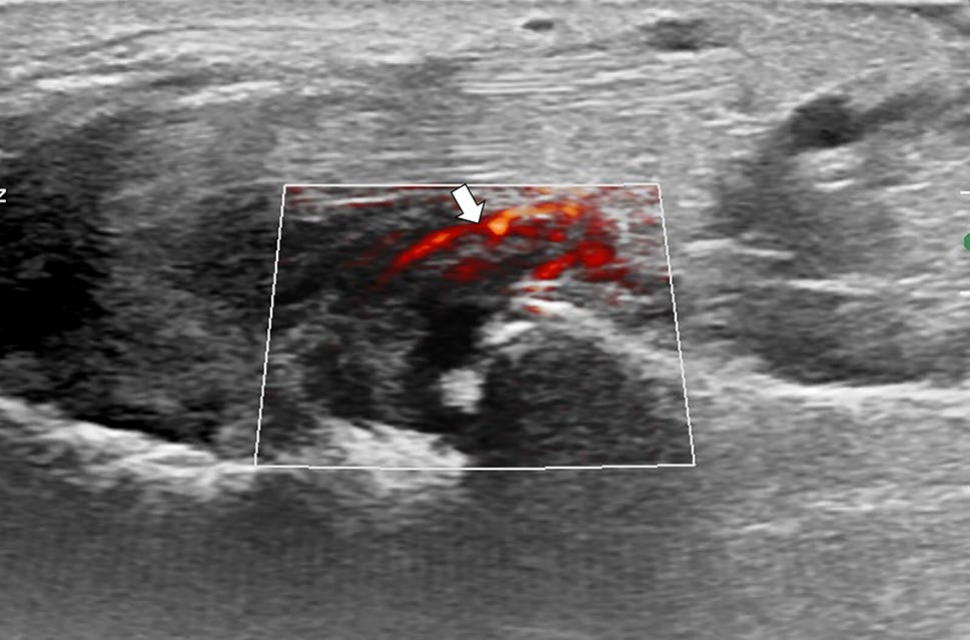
Increased second-degree synovial blood flow revealed in ultrasonography of the radiocarpal joint and intercarpal joint on the palmar side
